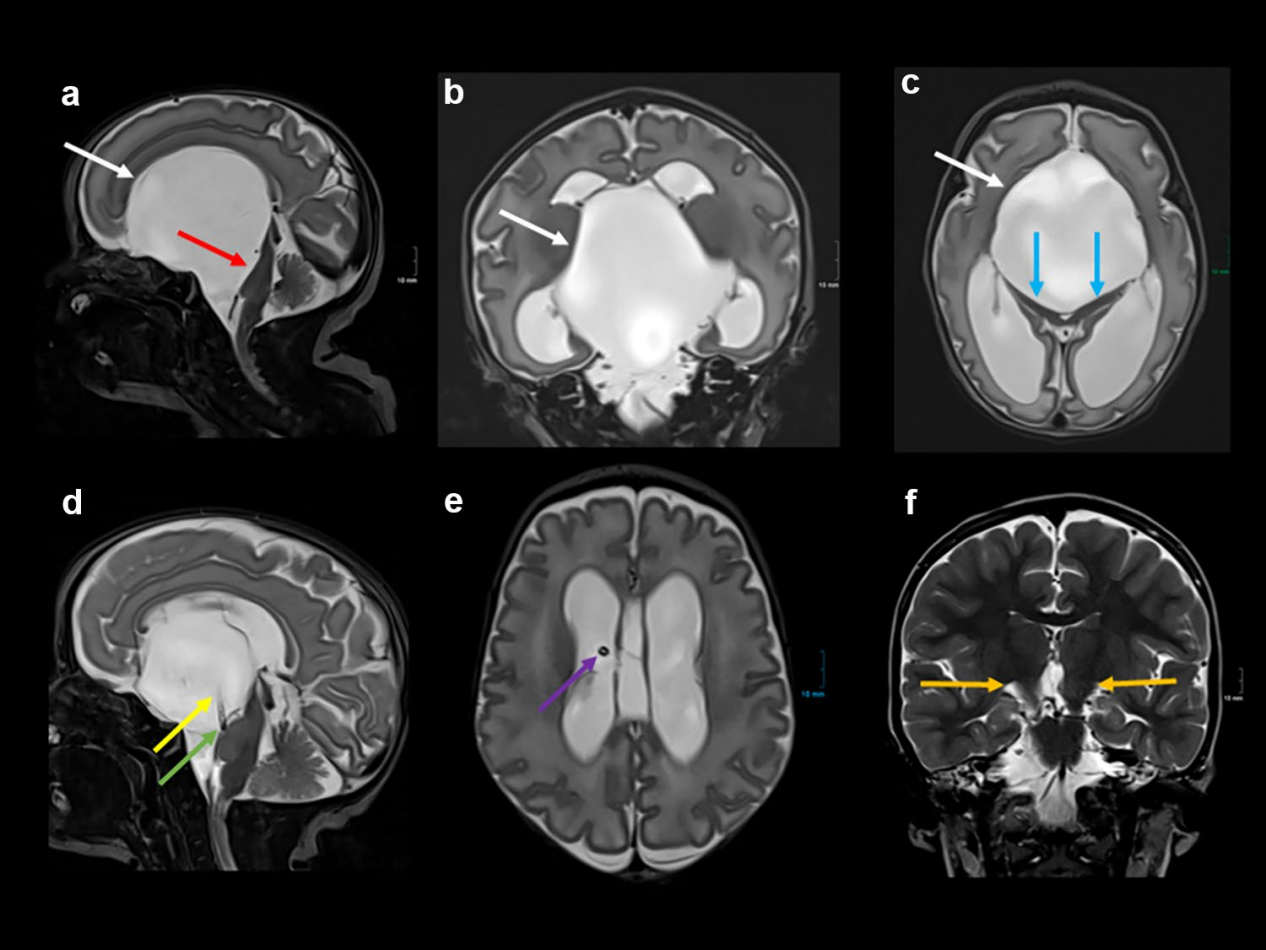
a–c Postnatal MRI (T2 TSE) in sagittal (a), coronal (b), and axial (c) orientations: large midline cystic formation (white arrows) with compression of the brainstem (a, red arrow) and the cerebral peduncles (c, blue arrows) causing significant hydrocephalus. d, e MRI (T2 TSE) in sagittal (d) and axial (e) orientations 1 month after endoscopic fenestration of the cyst. The implanted internal shunt catheter connects the cyst (d, yellow arrow) with the prepontine cistern (d, green arrow) and the lateral ventricle (e, purple arrow). Consecutive reduction of cyst size and hydrocephalus. f MRI at the age of 4 years (T2 TSE in coronal orientation; with friendly approval of Dr. Hundt, Radiology Munich Nymphenburg): subtotal regression of the cyst and no hydrocephalus with VP-Shunt (not shown), some asymmetry of the cerebral peduncles (orange arrows)
